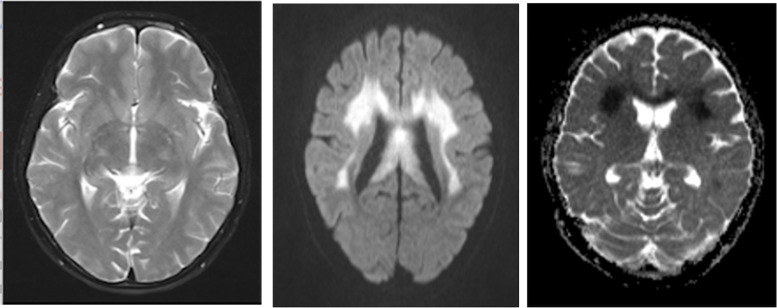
T2W FLAIR MRI sequence showing hyperintensities and corresponding diffusion-weighted imaging showing diffusion restriction involving bilateral central semi-ovale, corona radiata, posterior limb of internal capsule and also genu, body, and splenium of corpus callosum
